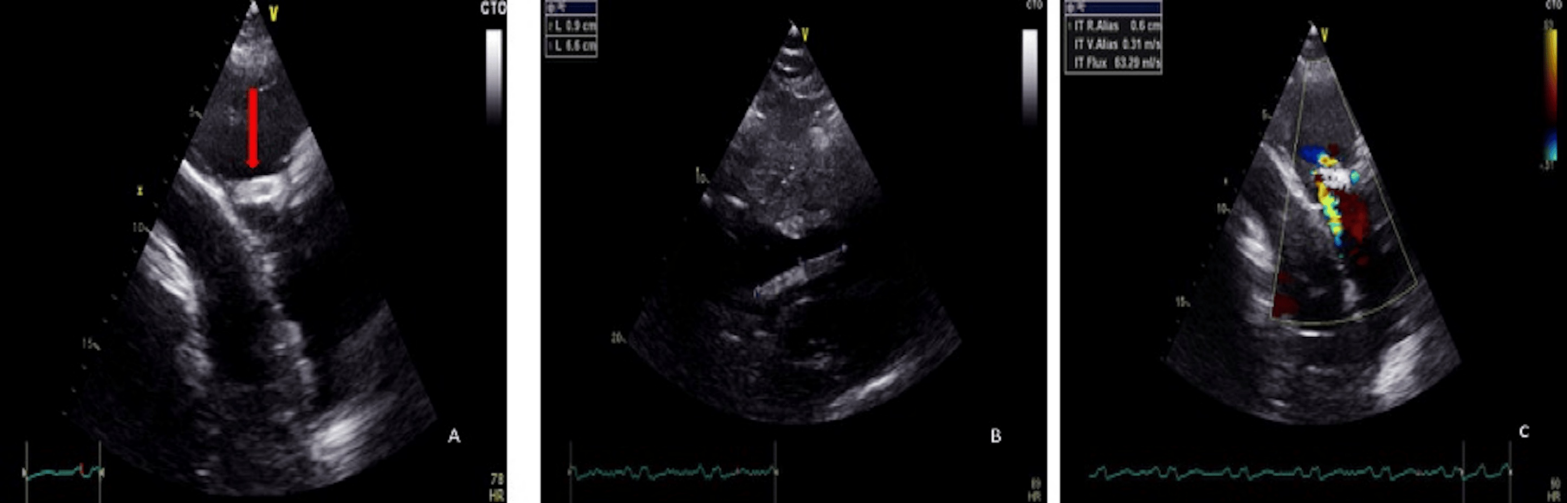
Transthoracic echocardiographic views. (A) Parasternal long-axis view showing a linear echogenic structure (red arrow) extending into the right ventricle, compatible with the venous stent. (B) Apical four-chamber view highlighting the stent embedded near the tricuspid subvalvular apparatus. (C) Color Doppler view revealing mild tricuspid regurgitation.
